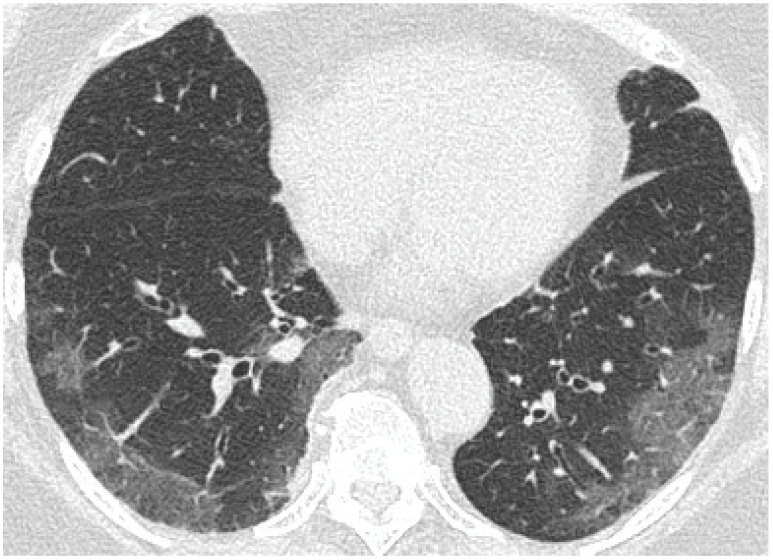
Axial plane of non-enhanced CT reveals ground glass opacities in subpleural distribution in lower lobes.
